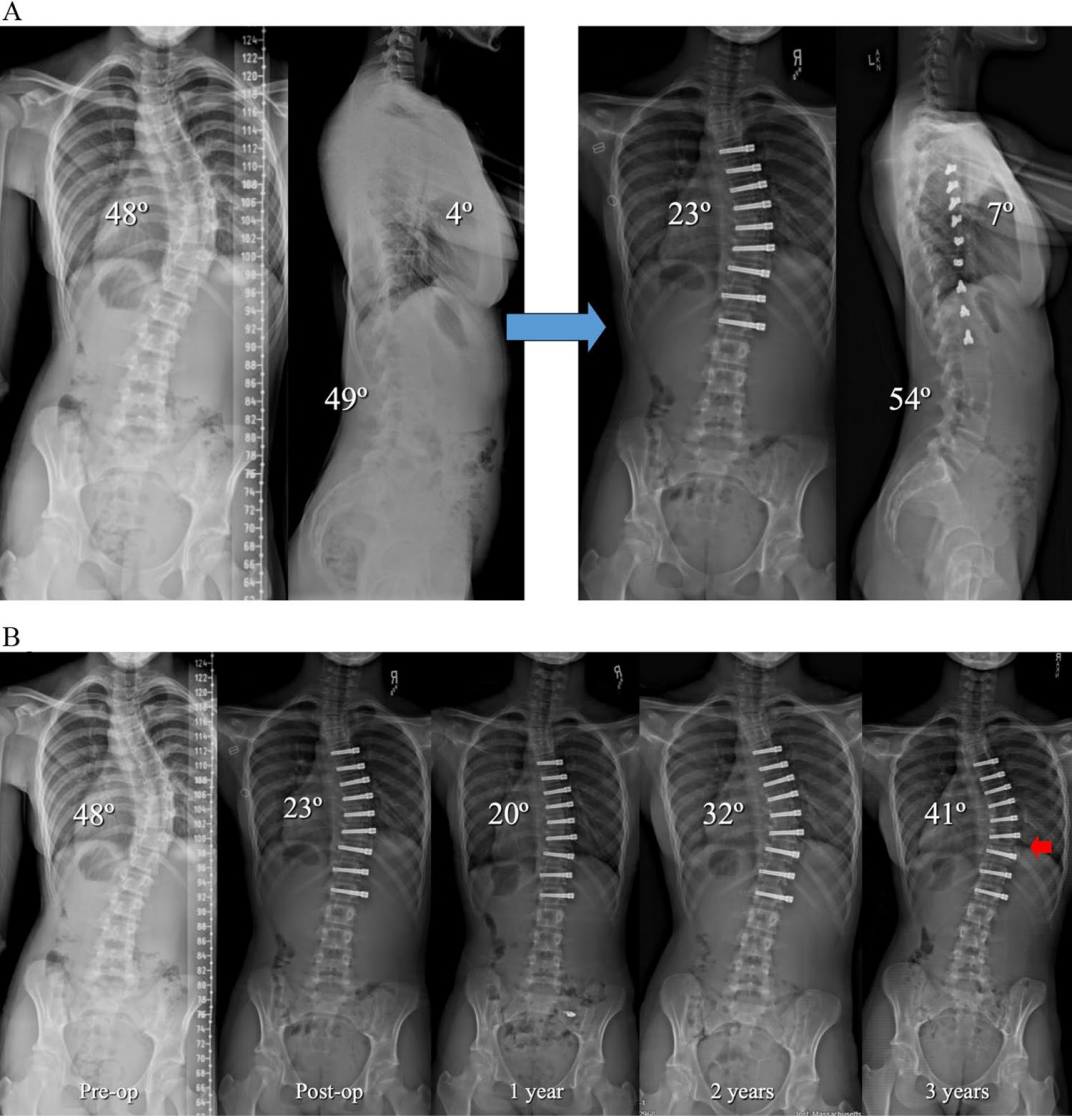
A Preoperative and postoperative posteroanterior and lateral radiographs of a 13 + 1 year-old premenarchal female with a Lenke 1AR curve pattern involving a 48º curve right T5-L1 at Risser 1 and Sanders 4. AVT T5-L1 resulted in initial curve correction from 48º to 23º with little change in the thoracic hypokyphosis. B Preoperative, postoperative, 1-year, 2-year, and 3-year posteroanterior radiographs demonstrated initial curve correction from 48º to 23º with subsequent improvement to 20º with growth modulation in the first year. A 12º loss of correction was evident at 2 years with another 9º loss of correction at 3 years. Though the 9º angle change at 3 years was correlated with an ISA change of 5º at T10,11, resulting in a consequential tether rupture (red arrow), the prior 12º loss of correction at 2 years did not correlate with an ISA change of ≥ 5º at any single level. This three sport athlete remains active without symptoms and without any interest in revision surgery at this time
